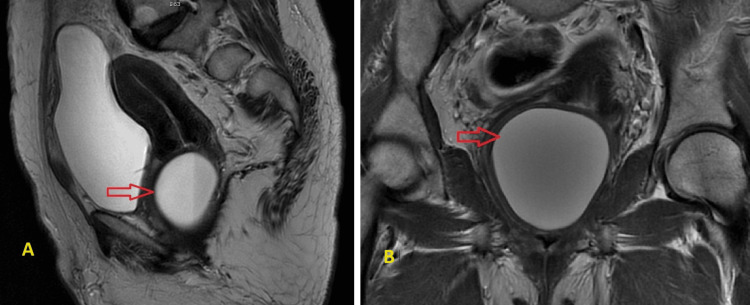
A: MRI midline sagittal view showing a well-defined thin-walled cystic lesion (red arrow mark) of size 6.6 x 5 x 5.5 cm along the posterior aspect of the mid and lower third of the vaginal wall. B: MRI coronal view showing the cyst (red arrow) compressing the vaginal canal anteriorly and abutting the rectum posteriorly.
